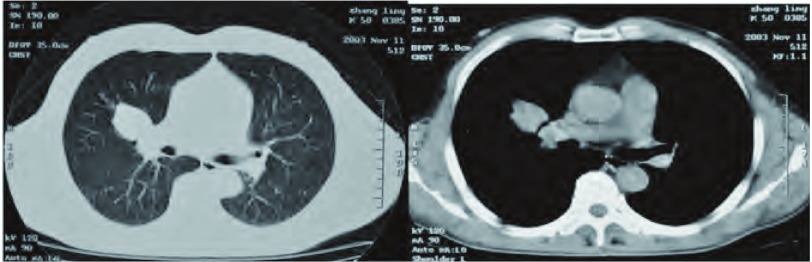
CT检查（2003年11月）：右上叶近肺门处软组织密度灶2.6 cm×3.1 cm，形态尚规则，密度均匀，边缘光整、无毛刺，与肺门、大血管境界清晰，CT值23 Hu。 CT scan of the chest (November, 2003): A tumor size is 2.6 cm×3.1 cm at superior lobe close the hilum of right lung but the boundaries between the big vessels of the gate is clear, the regular margin of the neoplasm could be observed without burr and the density is uniform, CT value is of 23 Hu.
